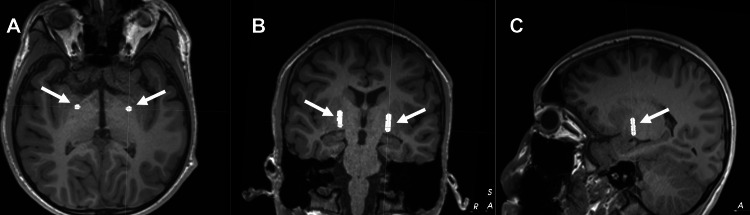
Depiction of bilateral single-electrode implantation sites generated from mapping of stereotactic target onto MRI for patient 2. (A) axial, (B) coronal, and (C) sagittal planes. Implantation sites are indicated by white arrows. MRI, magnetic resonance imaging
